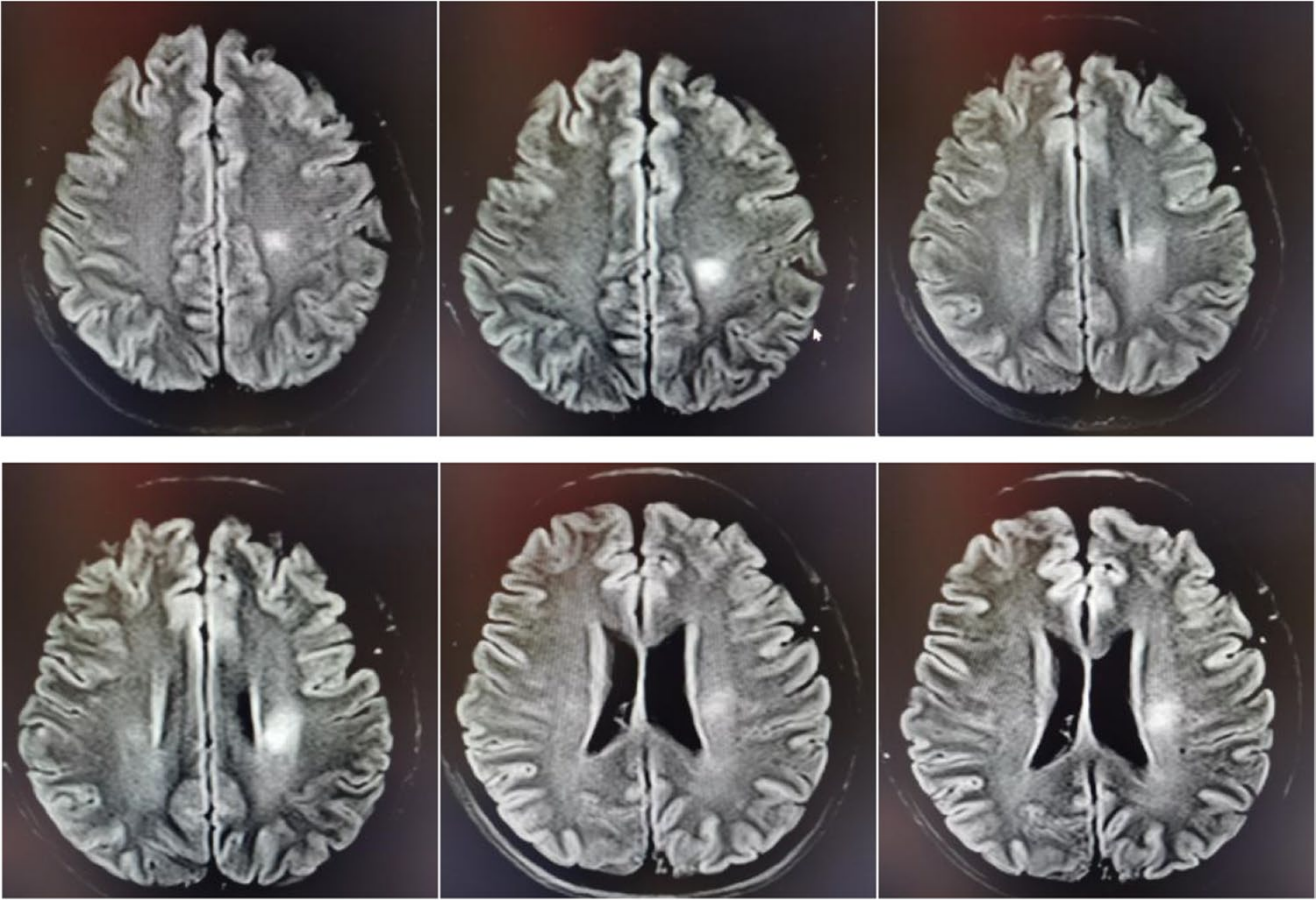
The MRI manifested abnormal signals in the left lateral ventricle and centrum semiovale on April 10, 2022
